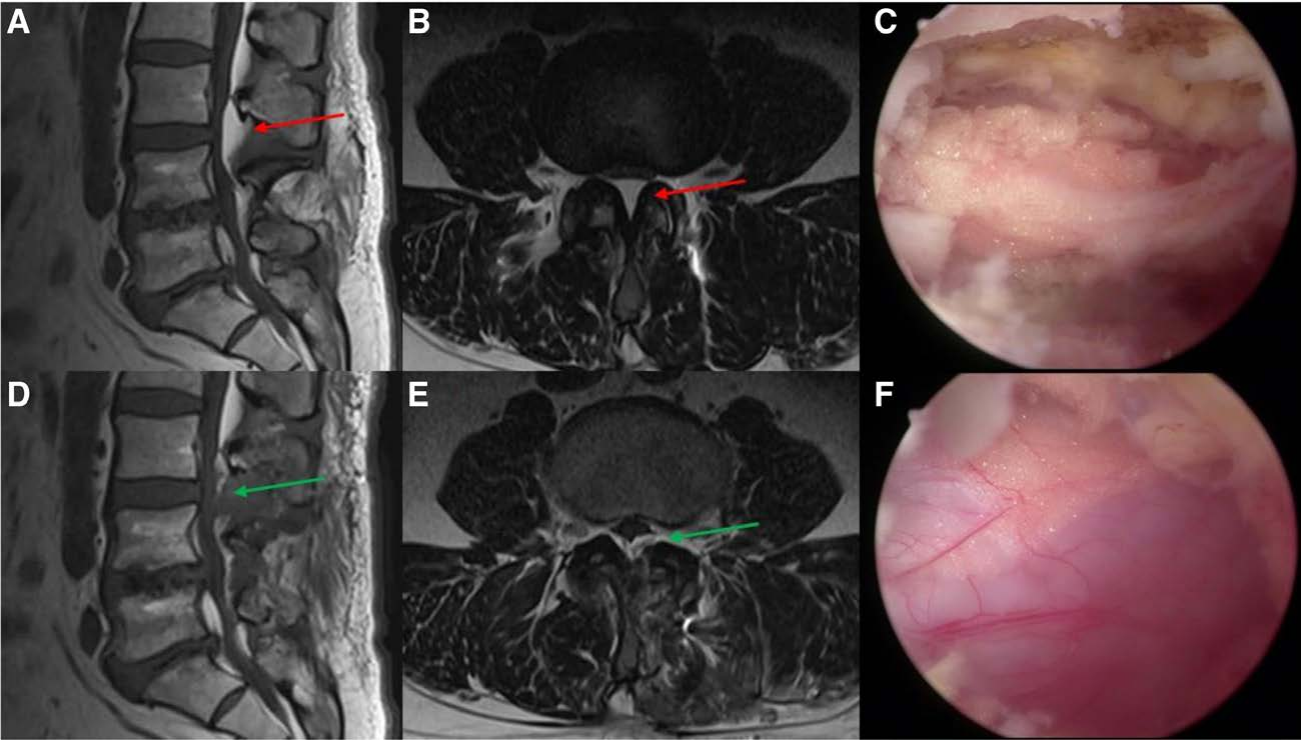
(A, B) Sagittal and axial MRI showed fat deposition (red arrow) compressing the dural sac. (C) Endoscopic image showing fat deposition in the spinal canal after laminotomy and flavectomy. (D, E) Sagittal and axial MRI showed a decompressed dural sac (green arrow) (F) The dural sac was completely decompressed after the fatty tissue was removed. MRI = magnetic resonance imaging.
